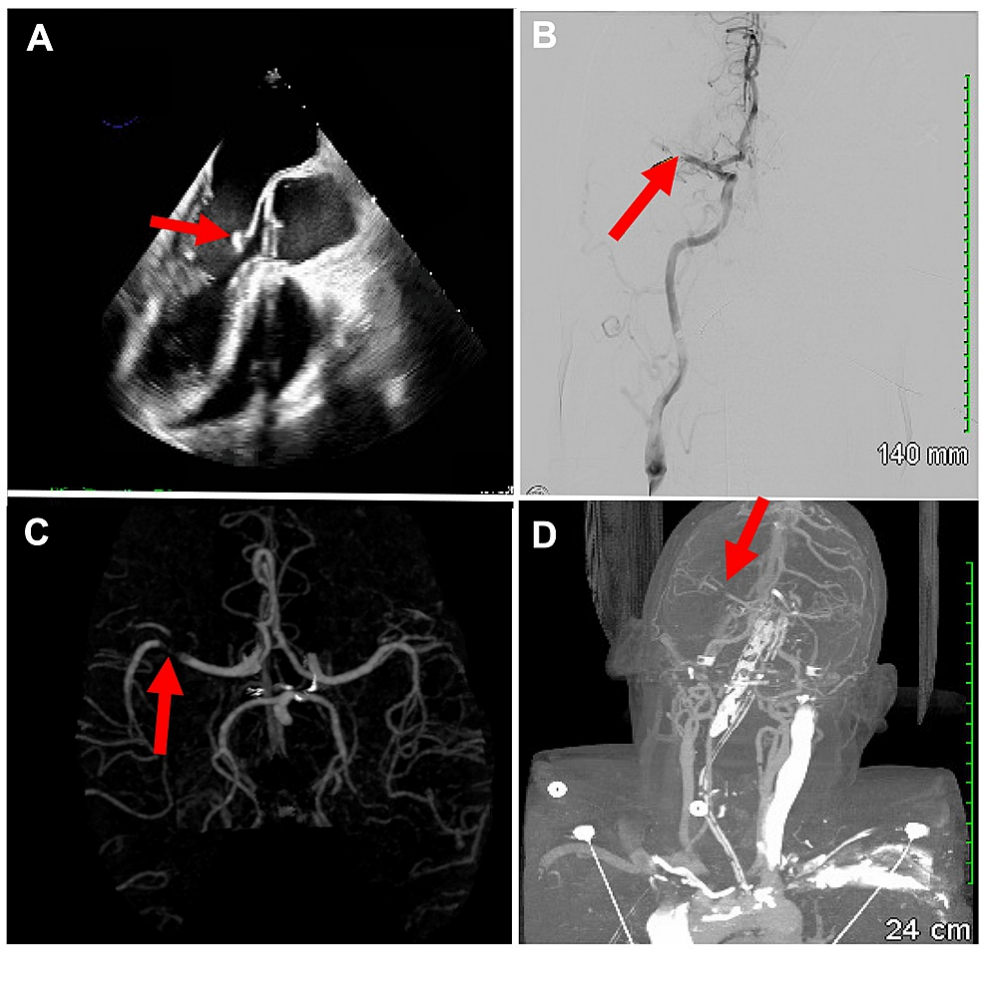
Acute left MCA occlusion due to marantic endocarditis A: Transesophageal echocardiogram showing a mass on the atrial side of the anterior leaflet of the mitral valve. B. Cerebral angiogram showing right M1 MCA occlusion. C & D: Computed tomography angiography (CTA) of the brain showing right M1 MCA occlusion.
